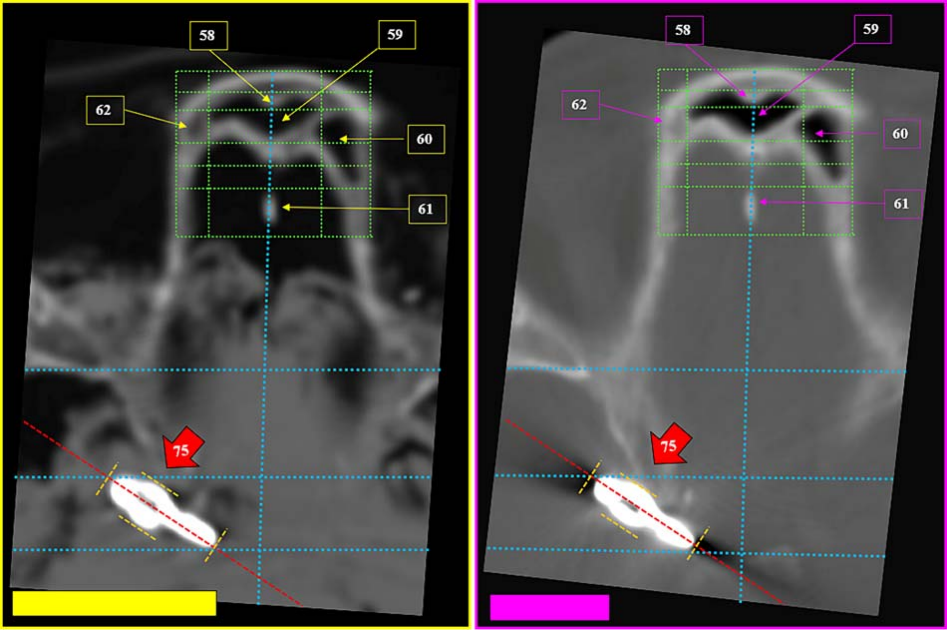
Details of the matching morphology and arrangement of the frontal sinus ethmoid recess (superior squares). Coinciding morphology and location of a device consistent with a vascular clip used in neurosurgical procedures (solid arrow). The oblique line represents the inclination of the clip in relation to the anatomical points (straight lines), highlighting the coinciding points between the antemortem (left) and postmortem (right) CT scans in axial sections.
